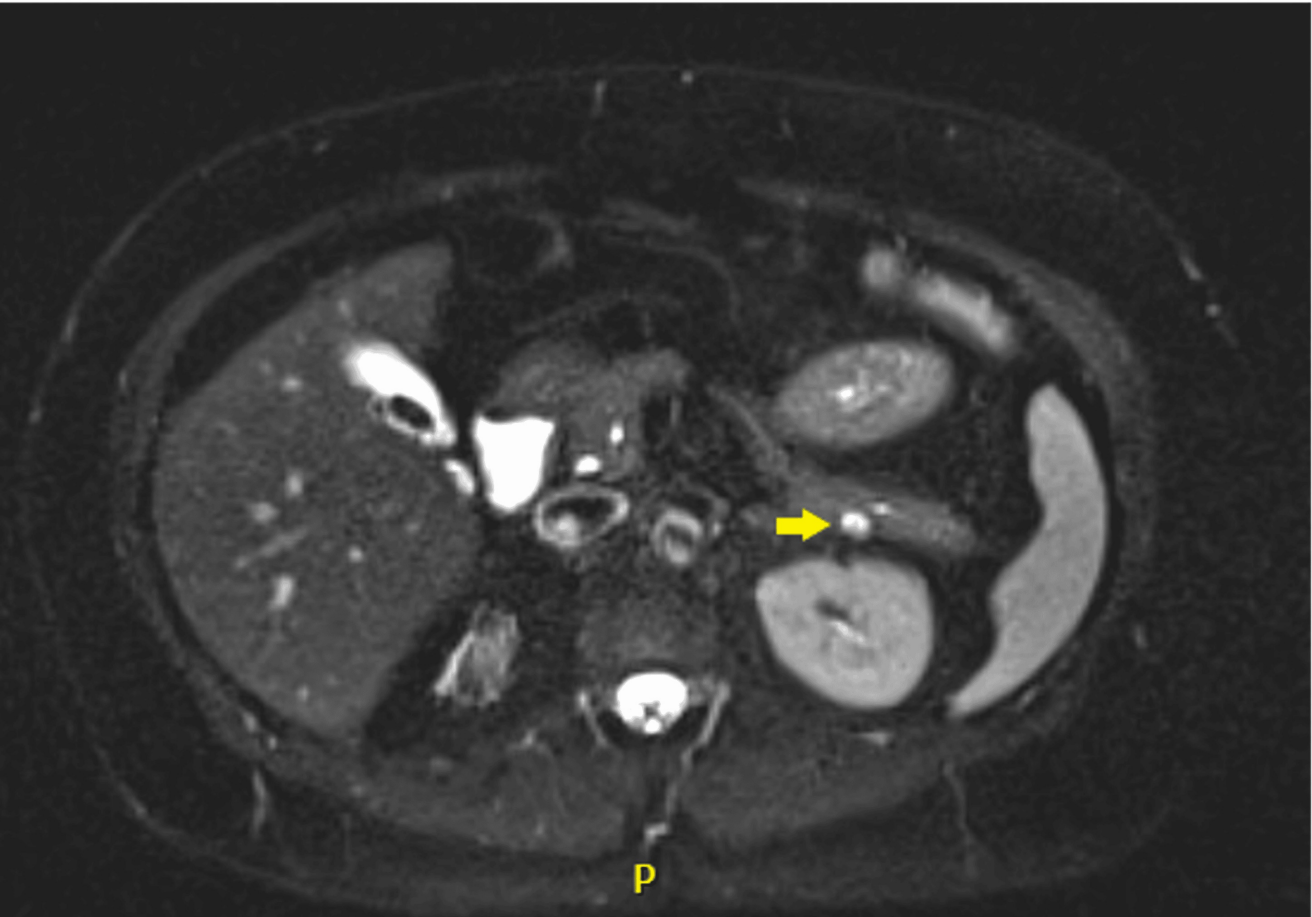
MRI performed in 2024 MRI of the pancreatic mass in 2024 showed increased growth at 13 x 10 mm (arrow). In comparison to the patient's previous CT scan in 2022, the increased growth and presentation of symptoms raised suspicion for a possible malignant lesion MRI: magnetic resonance imaging
